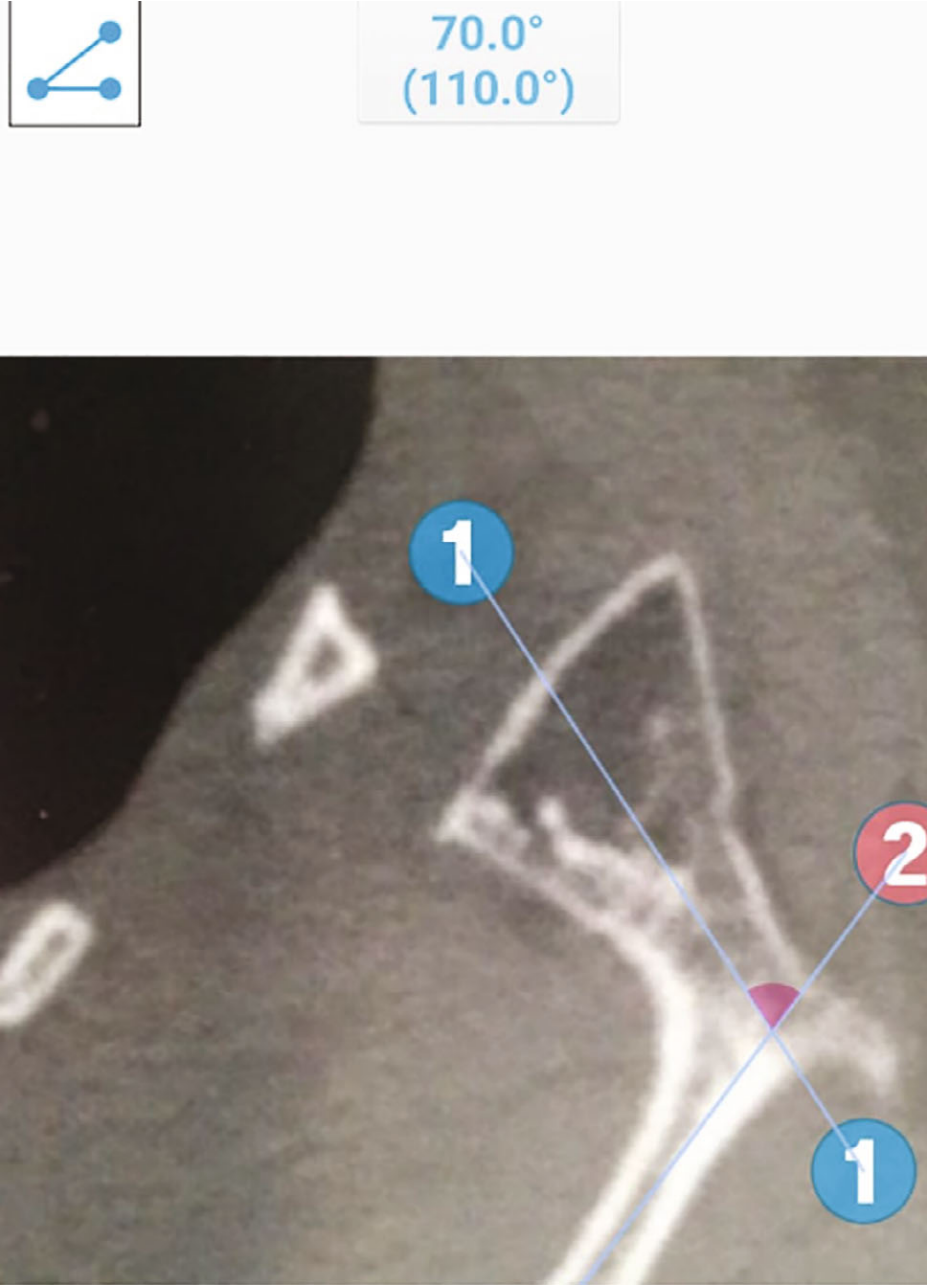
CT scan axial view of the right shoulder displays anteversion of the second glenoid of nearly 70°. Reproduced from http://www.BeeMed.com, with permission.
